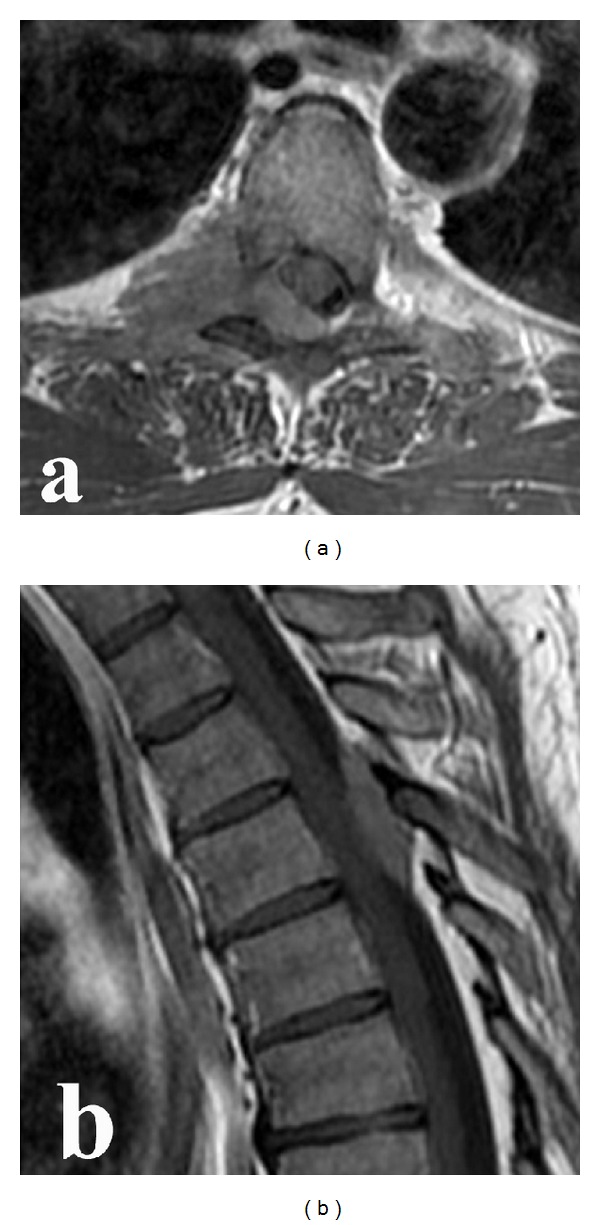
Preoperative axial (a) and sagittal (b) T1-weighted magnetic resonance imaging. Preoperative contrast enhanced magnetic resonance imaging scans showing a homogeneous enhanced dumbbell-shaped intra-extra-spinal mass at the D4–D6 level.
